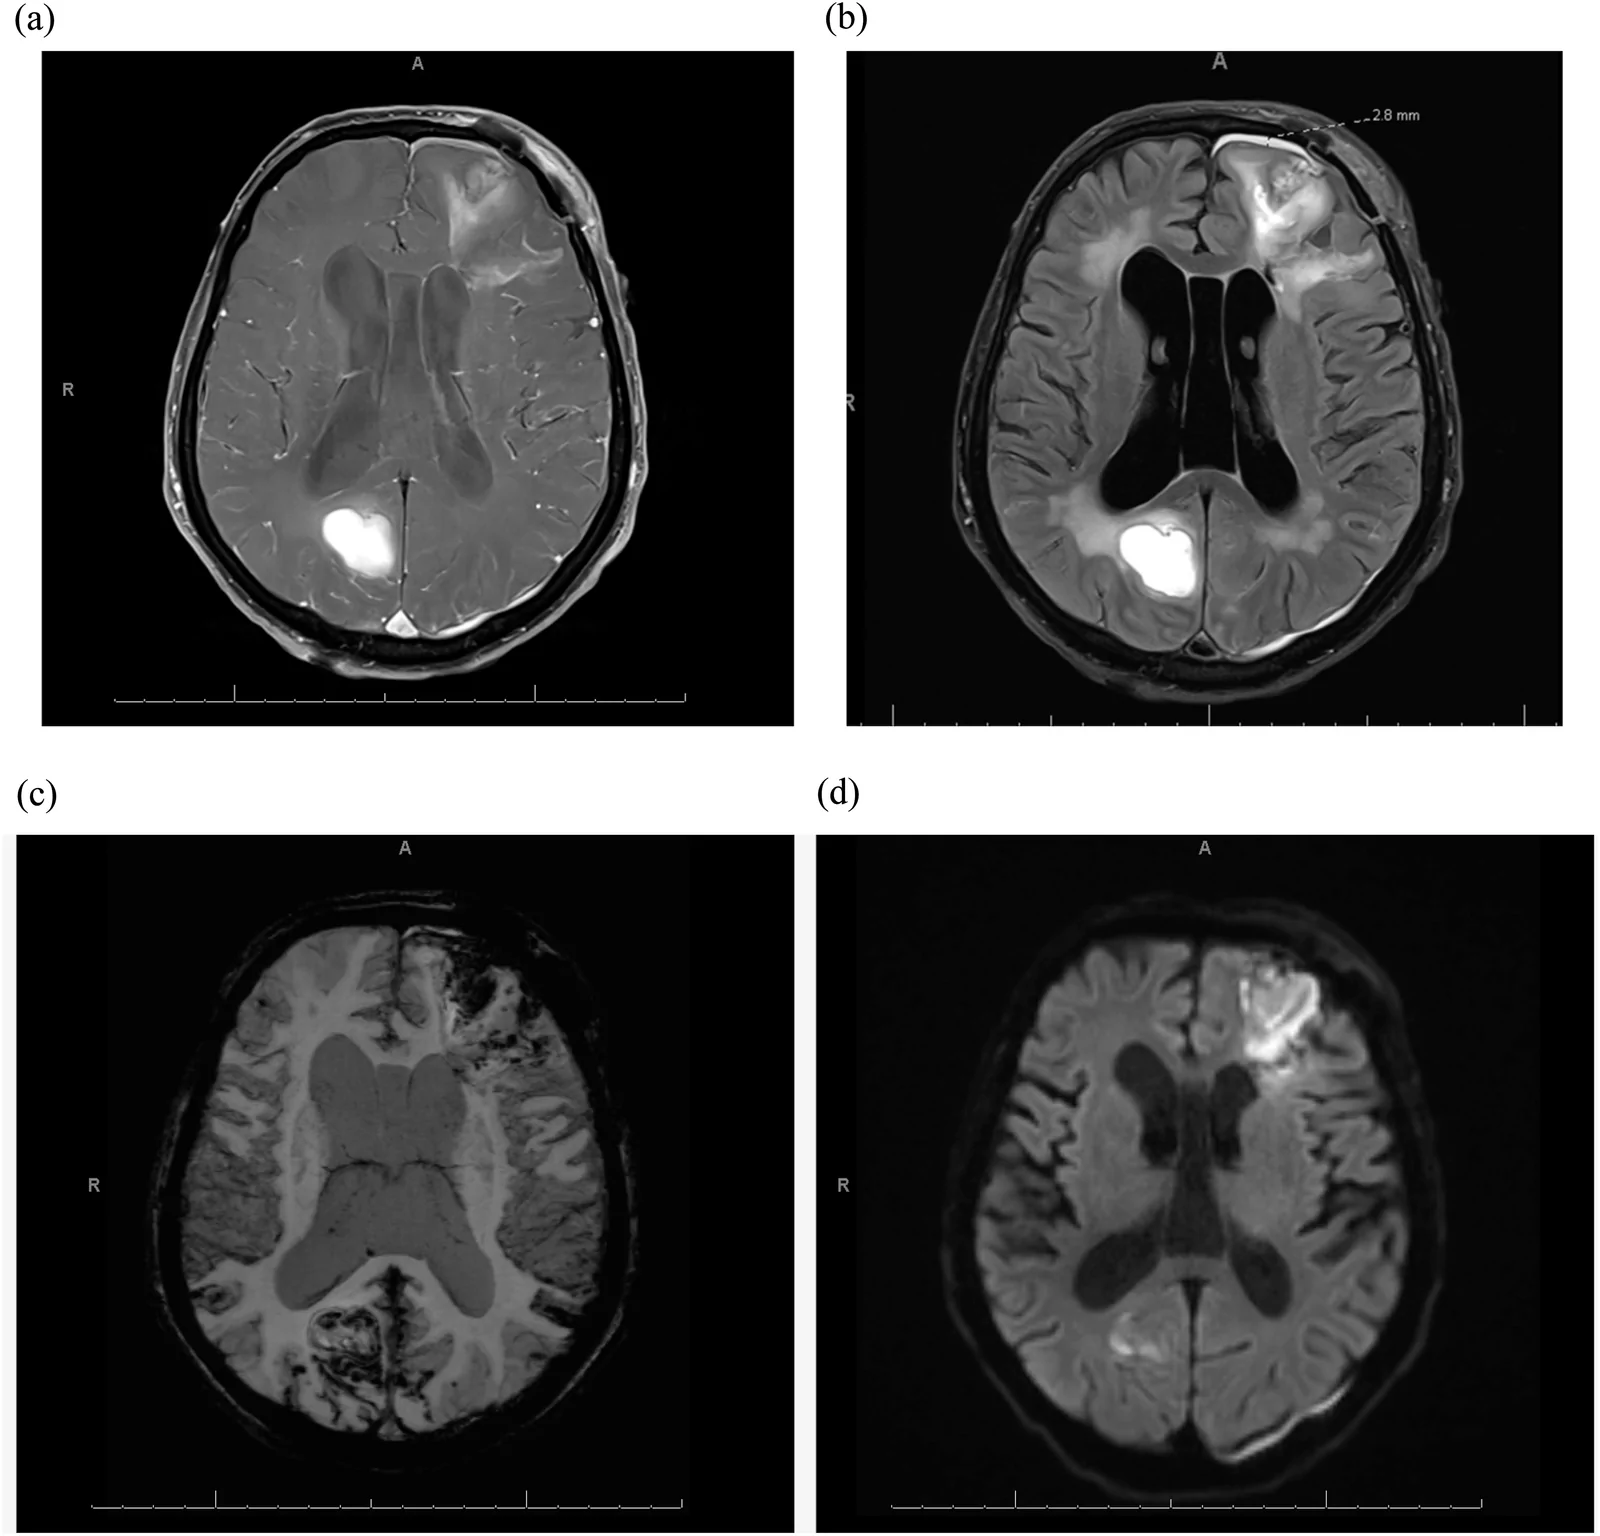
Follow-up MRI and susceptibility findings on 12/02/2025. (A) Follow-up axial post-contrast T1-weighted MRI demonstrated interval evolution of the left frontal intraparenchymal hematoma with cavity-margin enhancement. (B) Axial FLAIR MRI demonstrated increased bilateral convexity subarachnoid blood products compared with the prior MRI. (C) Axial susceptibility-weighted MRI demonstrated multifocal susceptibility effects corresponding to evolving intraparenchymal and extra-axial blood products. (D) Diffusion-weighted MRI demonstrated several new punctate areas of restricted diffusion involving the superior aspects of both cerebral hemispheres, right greater than left
